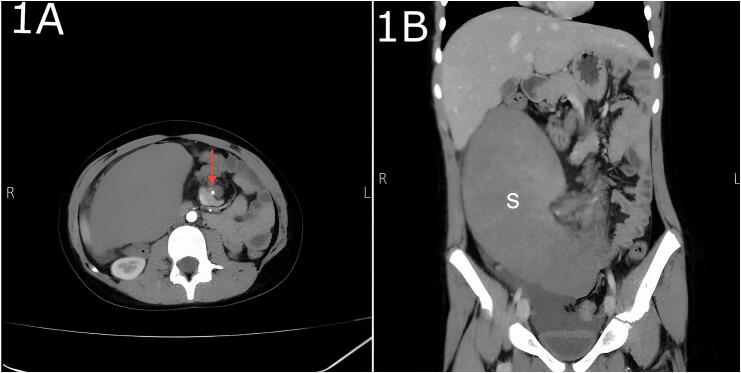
A: Axial contrast-enhanced CT image demonstrates a whorled appearance of the splenic vessels (red arrow), indicative of vascular torsion in a case of wandering spleen. This suggests splenic volvulus, a potential surgical emergency due to the risk of infarction. B: This contrast-enhanced coronal CT scan shows an ectopic spleen displaced into the right lower abdomen/pelvis, indicative of wandering spleen due to the absence or laxity of normal splenic ligamentous attachments. (For interpretation of the references to color in this figure legend, the reader is referred to the web version of this article.)
